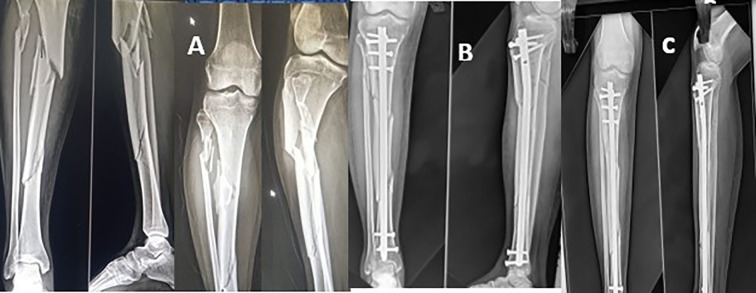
(A) Segmental fracture l tibia, (B) post op x-ray with suprapatellar nail and (C) six months follow up. Union of fracture.
